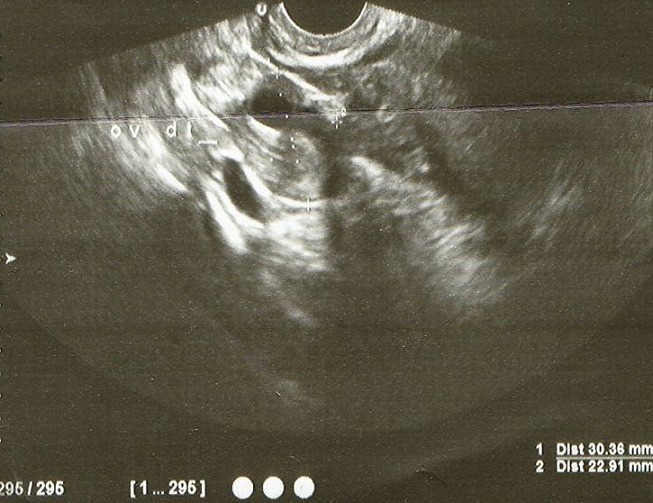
Ultrasound showed that the cyst is clearly separated from the ipsilateral ovary
